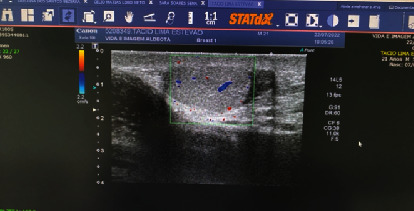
Ultrasound of the left hemiscrotum.
